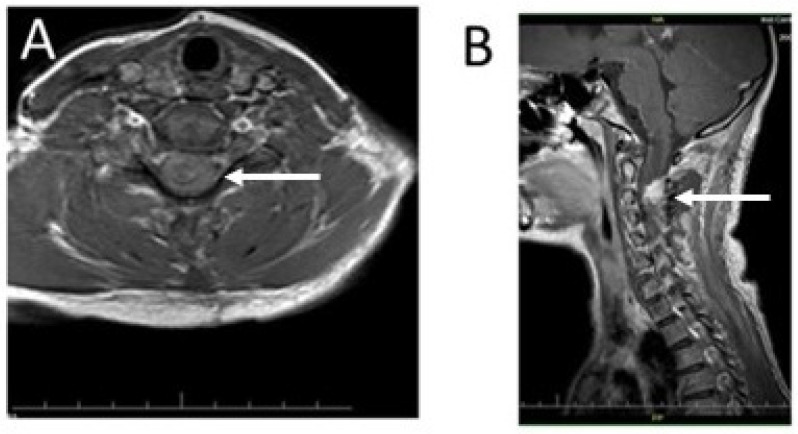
Imaging diagnostics of the patient’s cervical spine using T1-weighted MRI with contrast enhancement 48 h after the procedure: (A) an axial section of the cervical spine at the C2 level with clearly visible reduction of the syringomyelic cavity (arrow); (B) a sagittal scan of the cervical spine; the remaining part of the tumor mass in the cervical spine is visible as a hyperintense focus in contrast to the image of the spinal cord (arrow).
